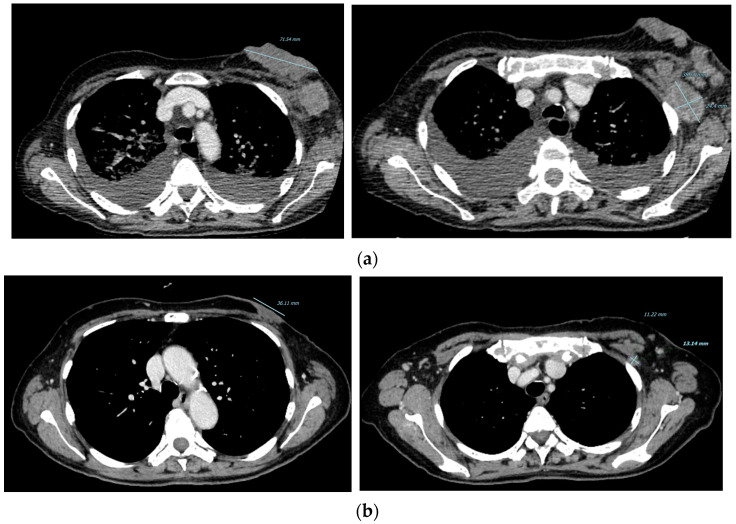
Baseline CT scan evaluation and subsequent time point: (a) CT scan March 2021, basal evaluation: left breast primitive lesion and left axilla lymphadenopathy; (b) CT scan November 2021: size reduction of left breast primitive lesion and left axilla lymphadenopathy.
